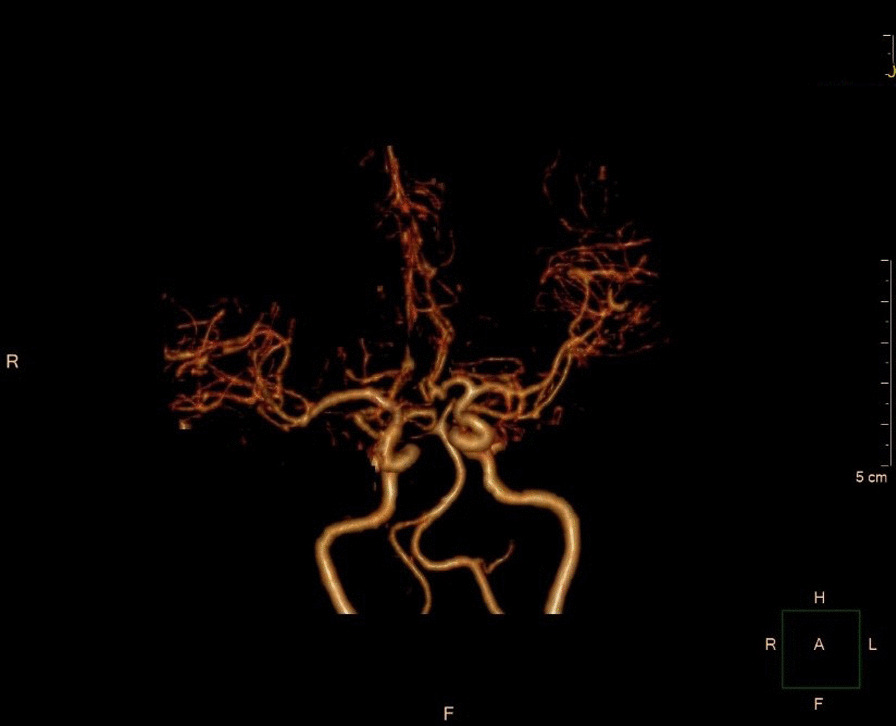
CTA results showed that there were no abnormalities such as embolism in the main cerebral artery
